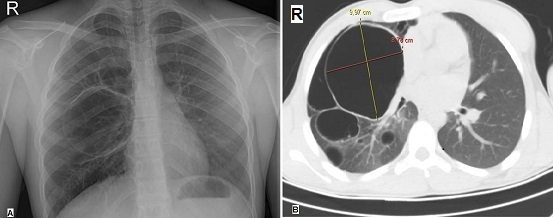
Multiple cystic lung disease. A) chest radiograph shows multiple doubtful cystic lesions in the right lung; B) chest CT scan shows a giant cyst in the right lung with multiple varying sizes and shapes air-filled cysts
